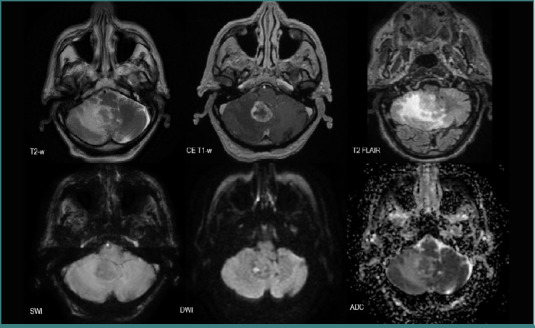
MRI brain acquisition of an NSCLC patient (adenocarcinoma). Highly edematous solid cerebellar lesion (high T2-w and T2-w FLAIR signals in the surrounding white matter) compressing the fourth ventricle. Discreet ‘blooming’ on SWI and diffusion restriction limited to a small area of the tumor (high DWI and low ADC). Peripheral contrast enhancement on the CE T1-W image suggests central necrosis.
